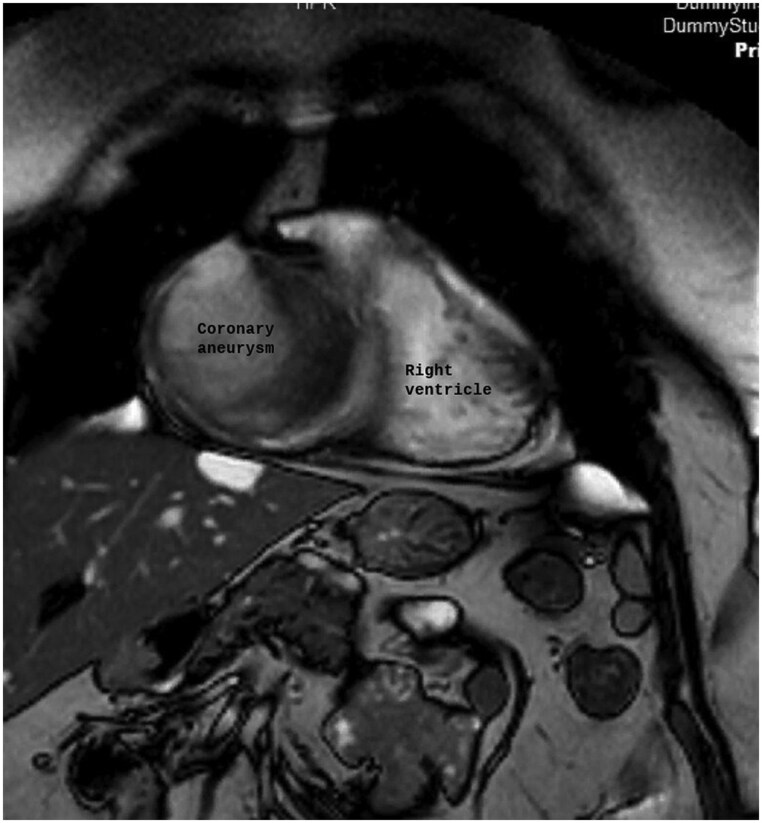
Coronal MRI view showing the right ventricle and, at the anatomical location of the right atrium, a large encapsulated rounded mass consistent with a giant right coronary artery aneurysm.
